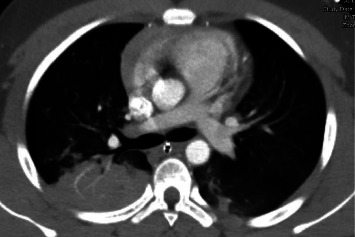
Axial view of CT chest scan showing bilateral alveolar ground-glass opacities with right upper lobe posterior segment consolidation.
